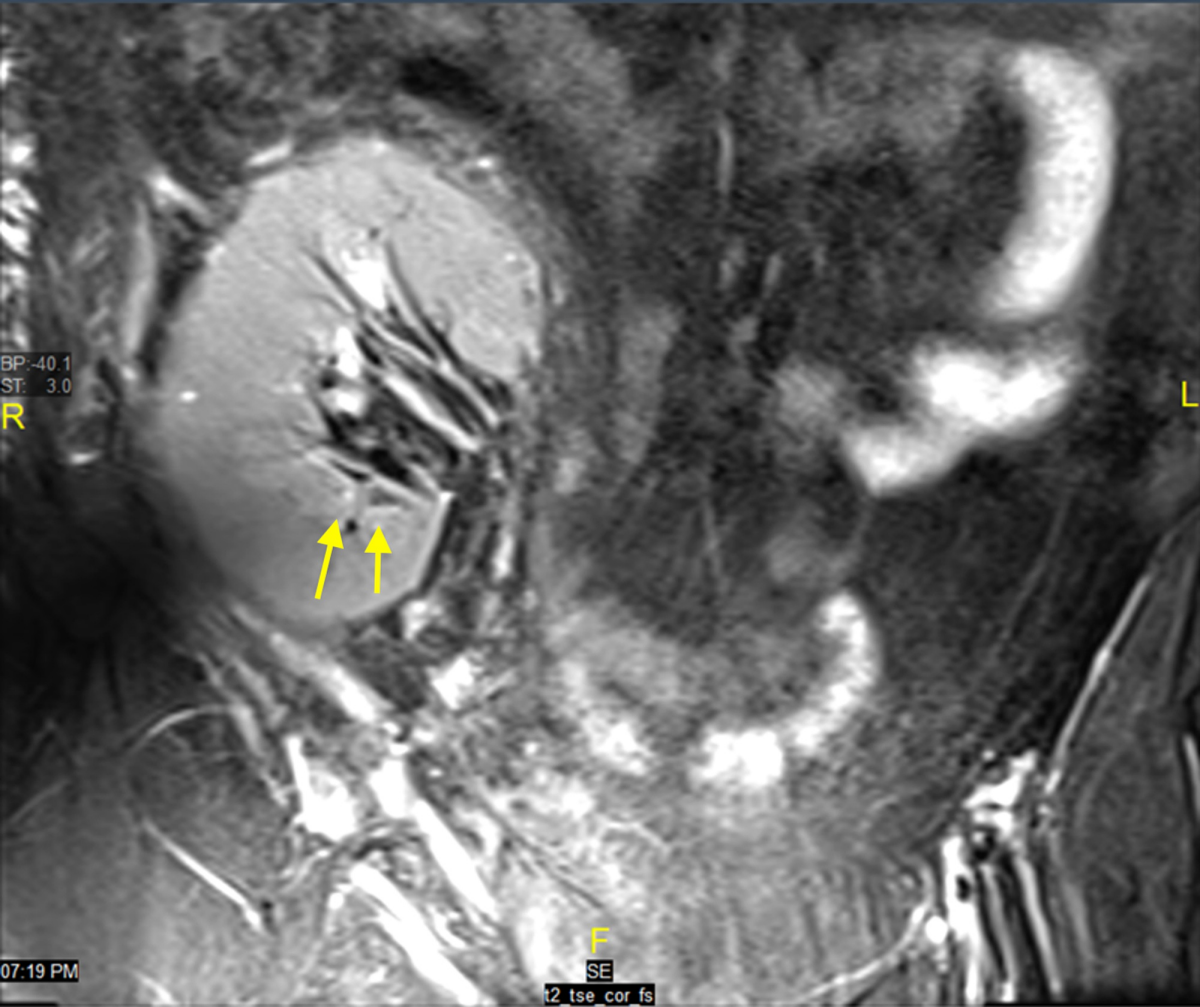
Magnetic resonance imaging (MRI) of the abdomen and pelvis The figure shows mild to moderate hydroureteronephrosis with the presence of calyceal clubbing and tiny cystic areas around the calyces, especially in the lower pole (yellow arrows). Multiple small parenchymal T2 bright cystic regions were also identified with few such areas demonstrating restricted diffusion, suggesting parenchymal micro-abscesses. Differential diagnosis of the possible etiology is graft site candidiasis, actinomycosis, mycobacterium tuberculosis, *Citrobacter braakii*, and cytomegalovirus (CMV).
